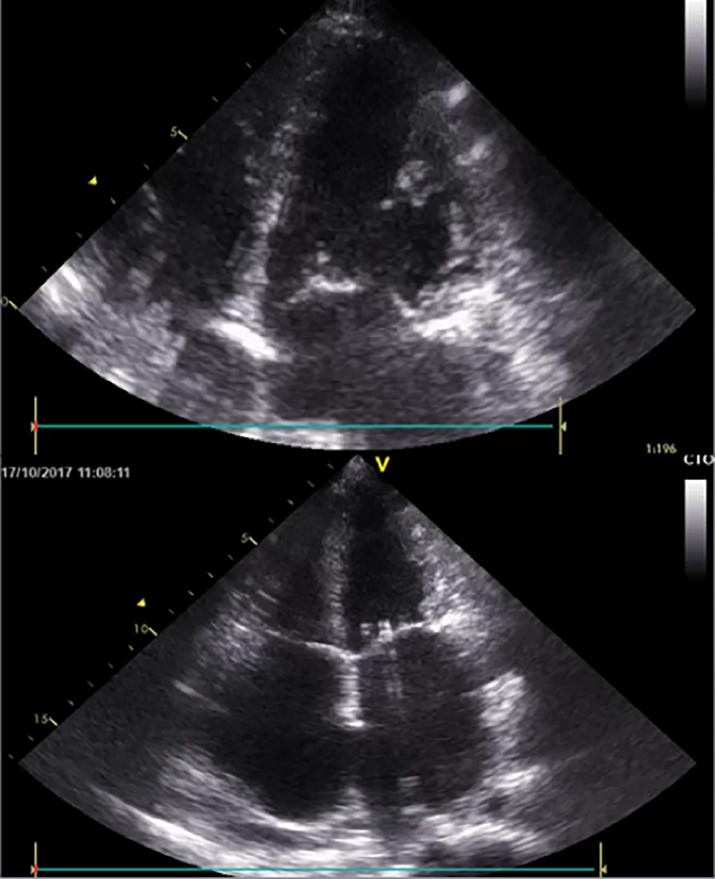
Echocardiography: Apical Four Chamber view, typical aspect of restrictive cardiomyopathy, severe biatrial enlargement with normal ventricular volume
